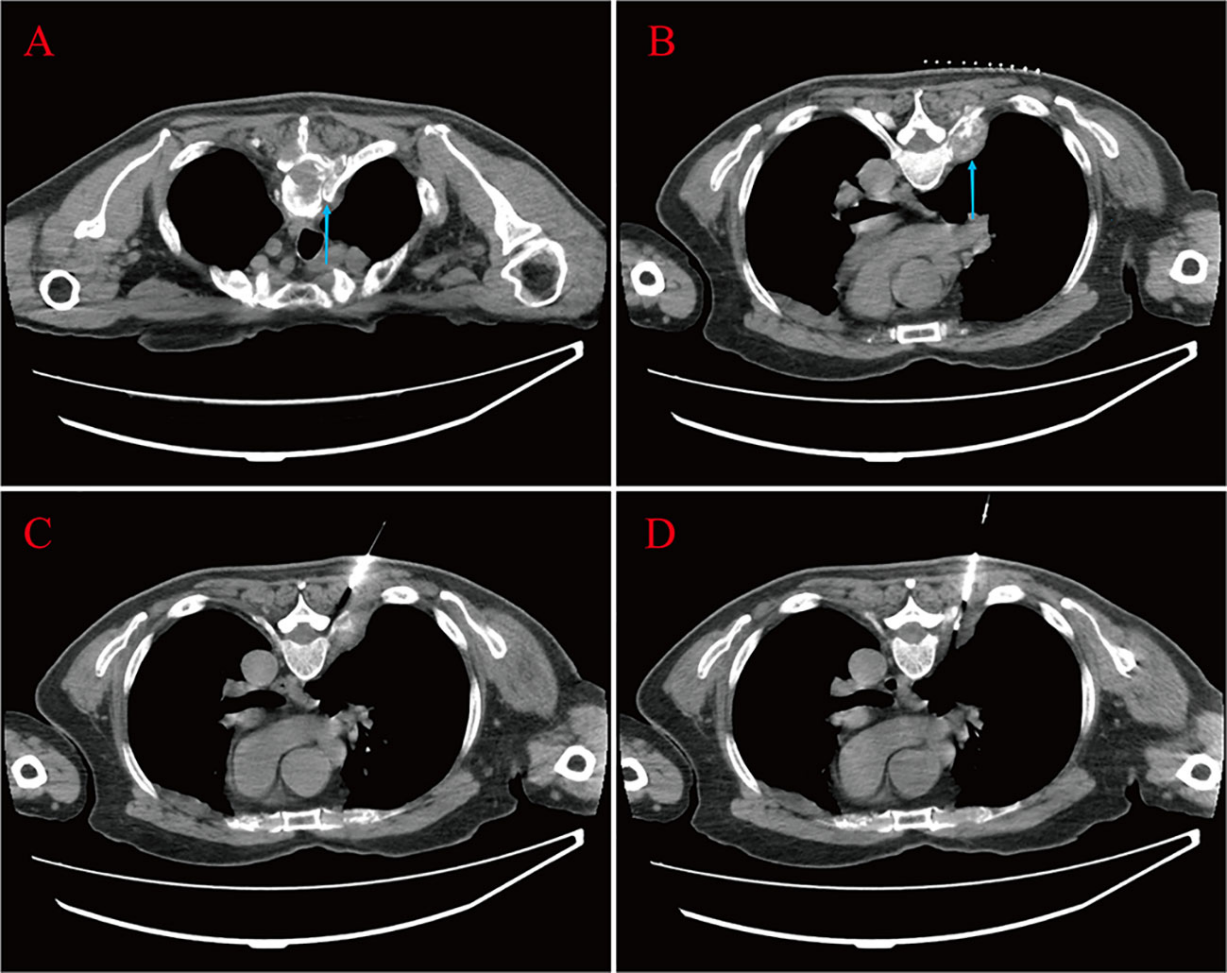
Bone destruction of the thoracic vertebrae and soft tissue mass formation at the root of the ribs are seen (blue arrow) (A, B). The patient lay prone on the CT examination bed; one strip of puncture tissue was taken and sent for analysis (C, D).
